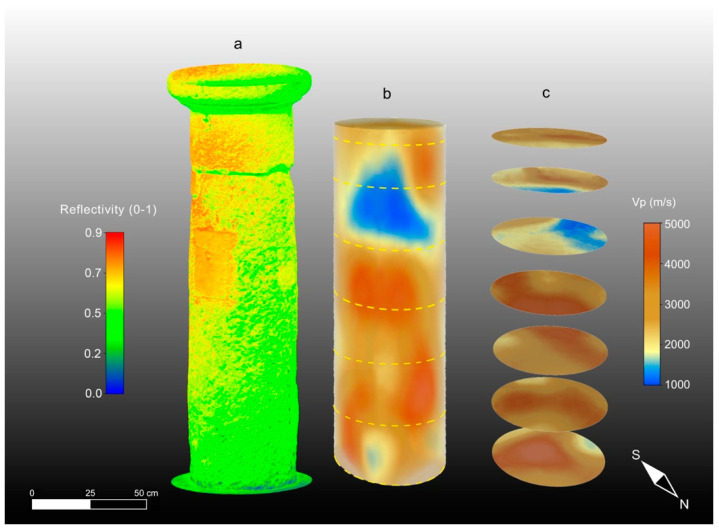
High-resolution 3D models of column 1. (a) TLS reflectivity model; (b) ultrasonic tomography; (c) tomographic slices.
